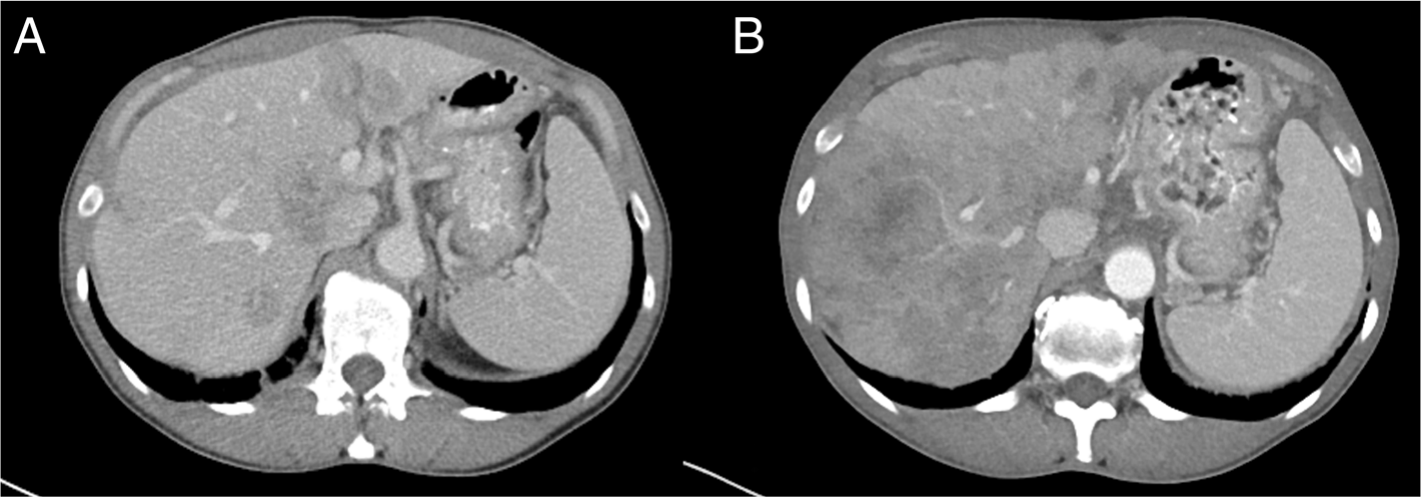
Image quality comparison in parenchymal liver lesions. Same patient in progressive disease. a Flash-CT: CTDIvol 14.40 mGy, DLP 1035 mGy · cm. b Force-CT: CTDIvol 13.35 mGy, DLP 946 mGy · cm
